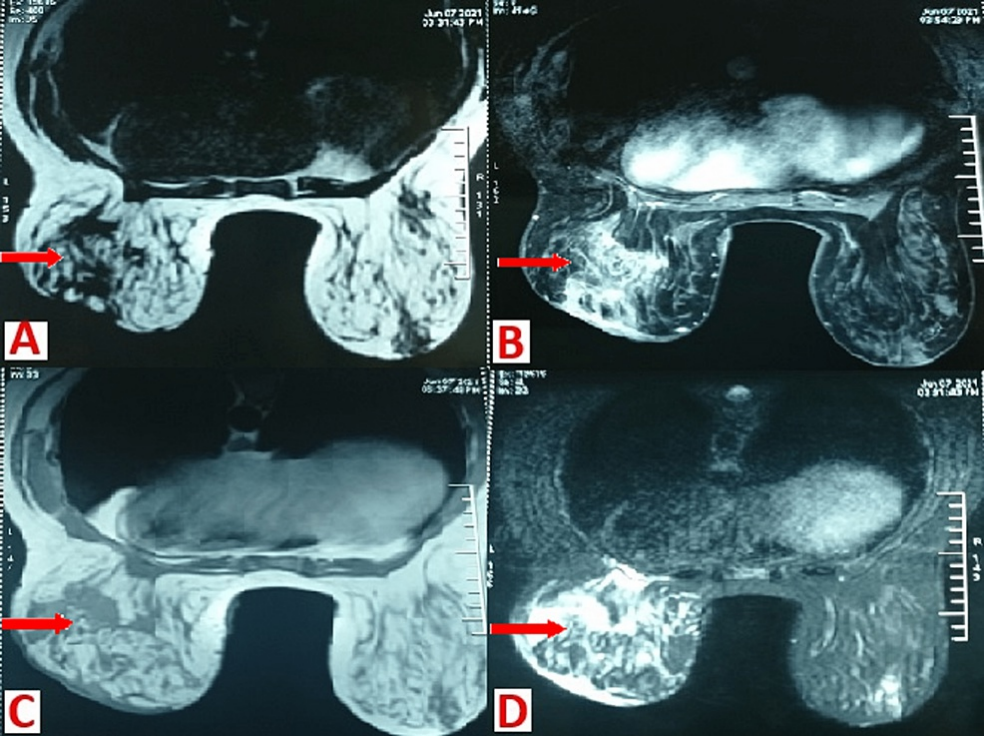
MRI scans. Axial slices of the left breast MRI T1 (A), T2 (B), T1 with injected gadolinium (C), injected T2 (D), showing a minimally limited infiltration of retro-mammillary region and lower quadrants, in ISO T1 signal, discretely heterogeneous T2 hypersignal, raising after injection, infiltrating forward the skin and the areolar plaque and back the deep muscular plane, classified BIRADS IVa.
